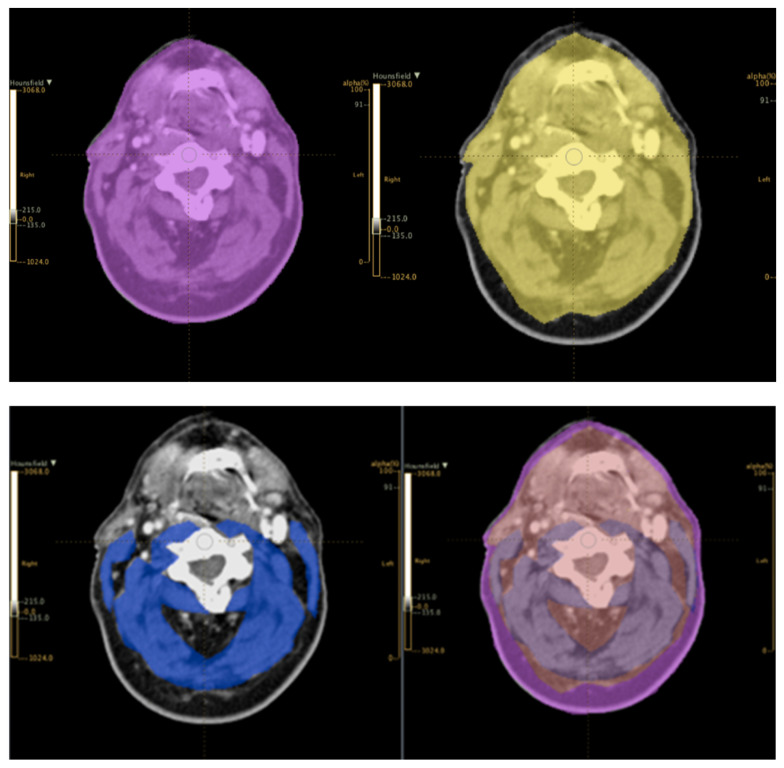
Example of a CT scan contouring at the level of C3. Above: contour of the skin profile on the left, contour of the subcutaneous fat on the right. Bottom: contour of the muscle profile on the left, three profiles shown simultaneously on the right.
